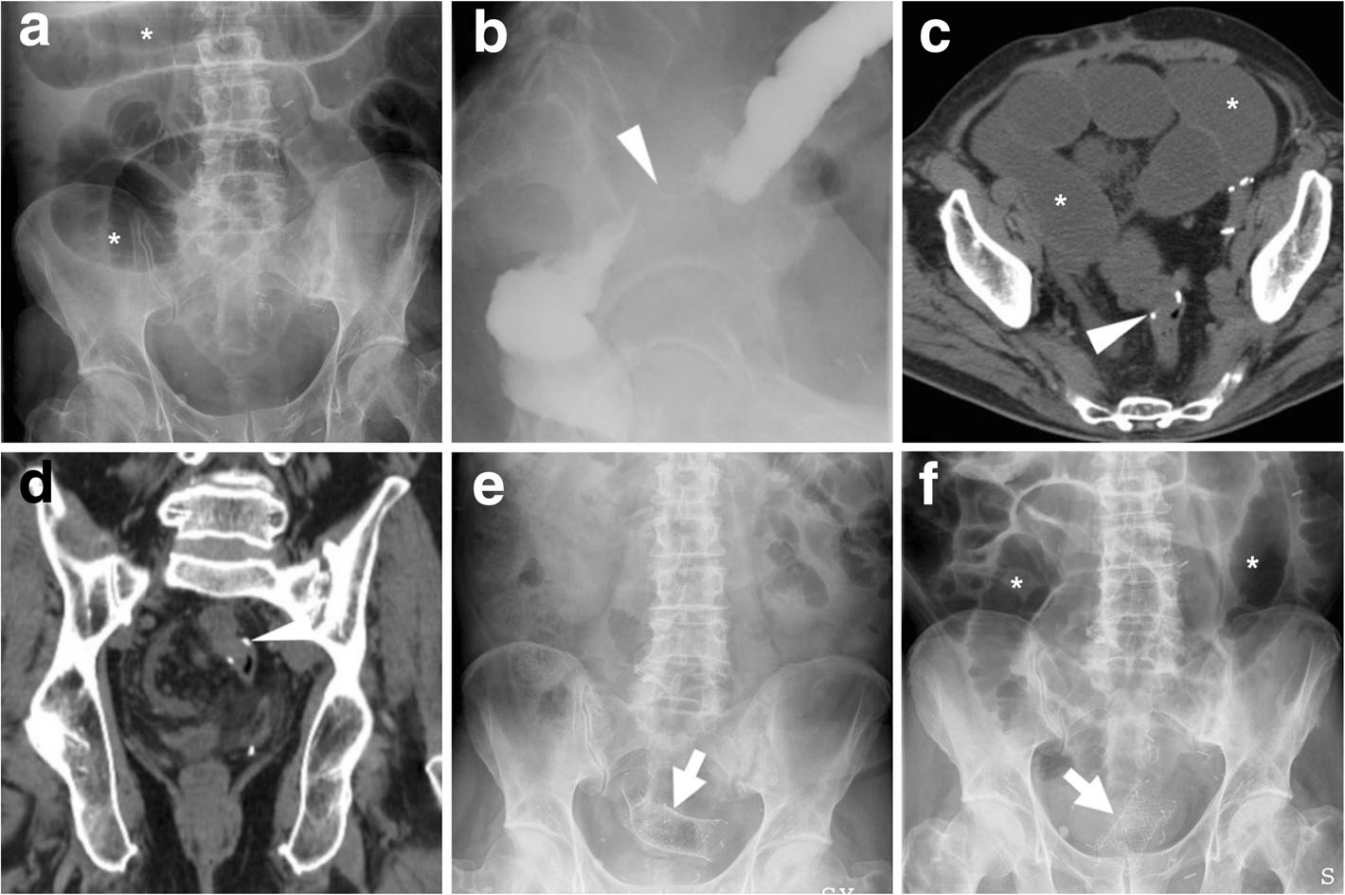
Stent management of a benign anastomotic stricture and SEMS displacement. In a patient with a history of resected sigmoid CRC, plain radiographic (a) shows LBO (*). Fluoroscopic contrast enema (b) and endoscopy confirm a tight stricture at the site of colorectal anastomosis (arrowhead in b) located 12 cm above the anal verge. Noncontrast CT (c, d) confirms fluid-filled dilated colon (*), without abnormal mural thickening or extraluminal mass at the anastomosis (arrowheads). A SEMS (thick arrows) was placed through the stricture (e). Unfortunately, LBO (* in f) ultimately recurred after SEMS displacement
